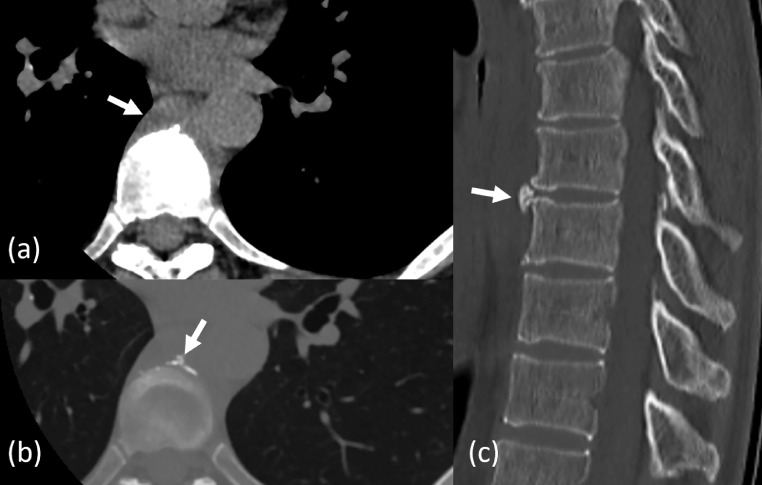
Computed tomography images of the Th6-9 vertebral bodies. (a) The axial view shows a soft tissue mass (arrow) extending ventral to the Th6-9 vertebral bodies. (b) The axial and (c) sagittal views of the bone condition reveal a polygonal high-density lesion (arrow) on the dorsal side of the mass and the ventral side of the Th8-9 vertebral bodies, respectively.
